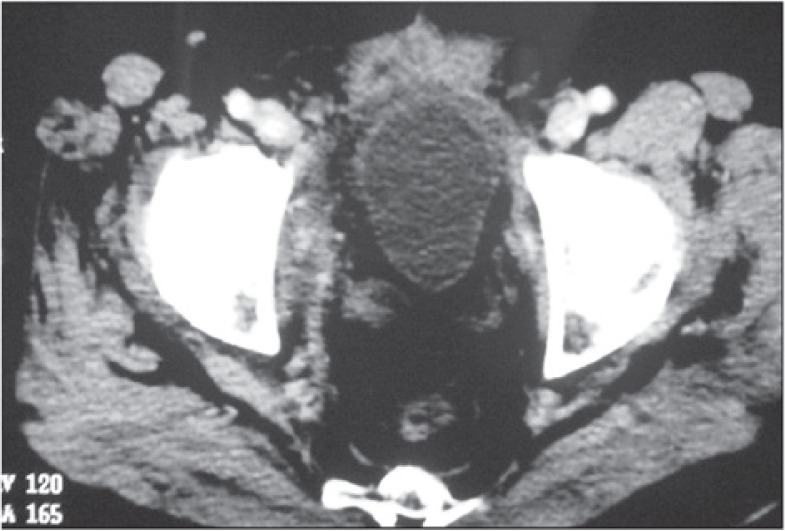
Computed tomography (CT) scan exhibiting no evidence of recurrence, 24 months following the surgery.
